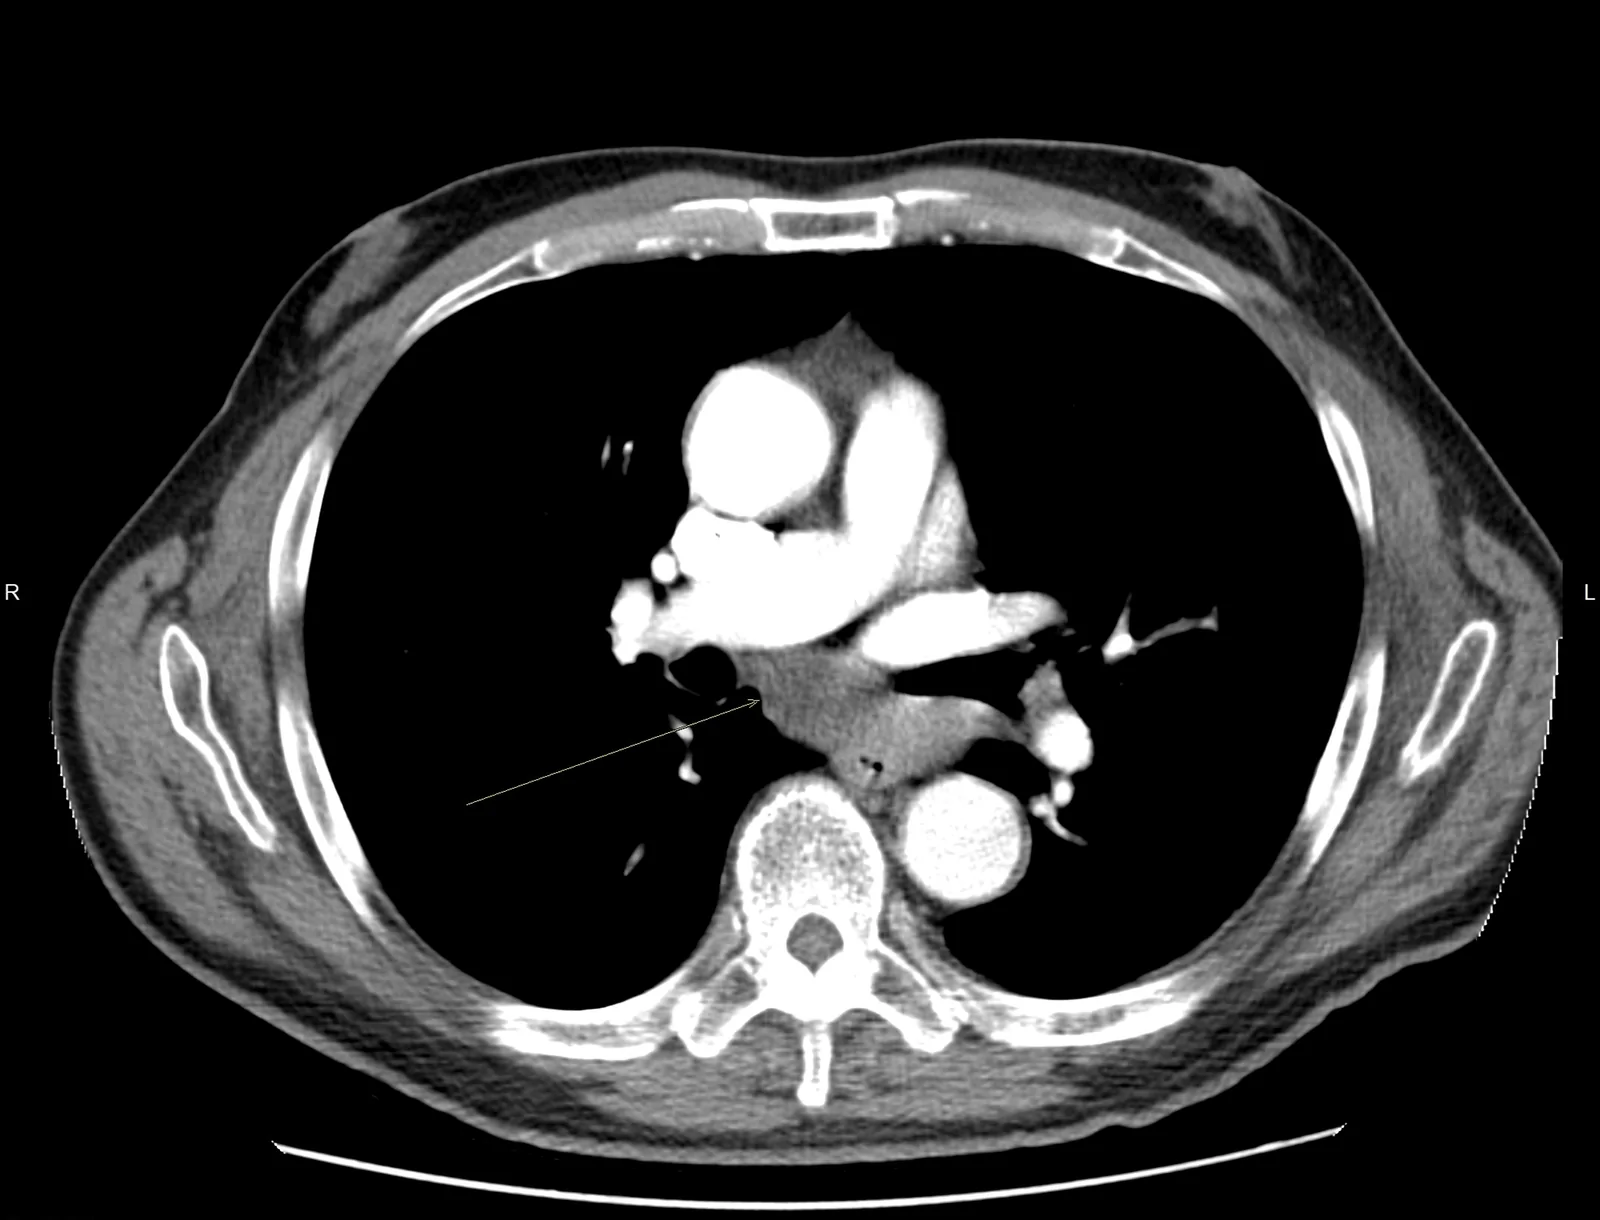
Chest CT demonstrating subcarinal lymphadenopathy Axial chest CT demonstrates subcarinal lymphadenopathy. The arrow indicates an enlarged subcarinal lymph node measuring approximately 2.4 × 3.4 cm CT: computed tomography
